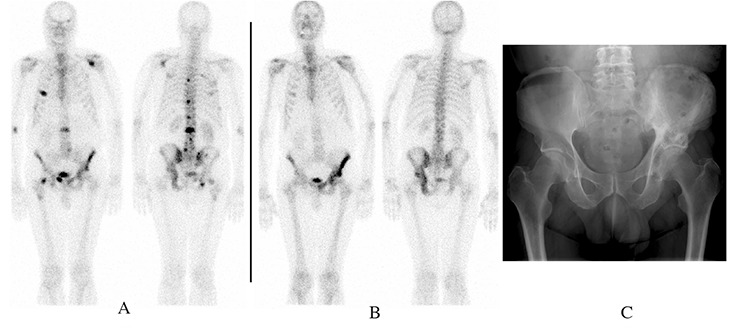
A) Whole-body bone scintigram demonstrates secondaries at multiple sites. Faint diffuse increased uptake is visible in the left hemi-pelvis
